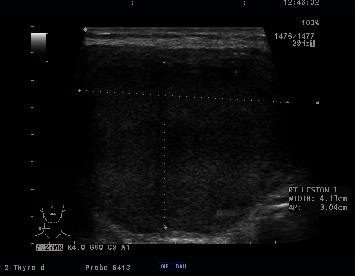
Ultrasound image at the time of presentation with neck pain and fever, showing the same thyroid nodule with increase in size measuring 4.95 × 4.13 × 3.04 cm and with interval development of isoechoic debris in the cystic fluid.
